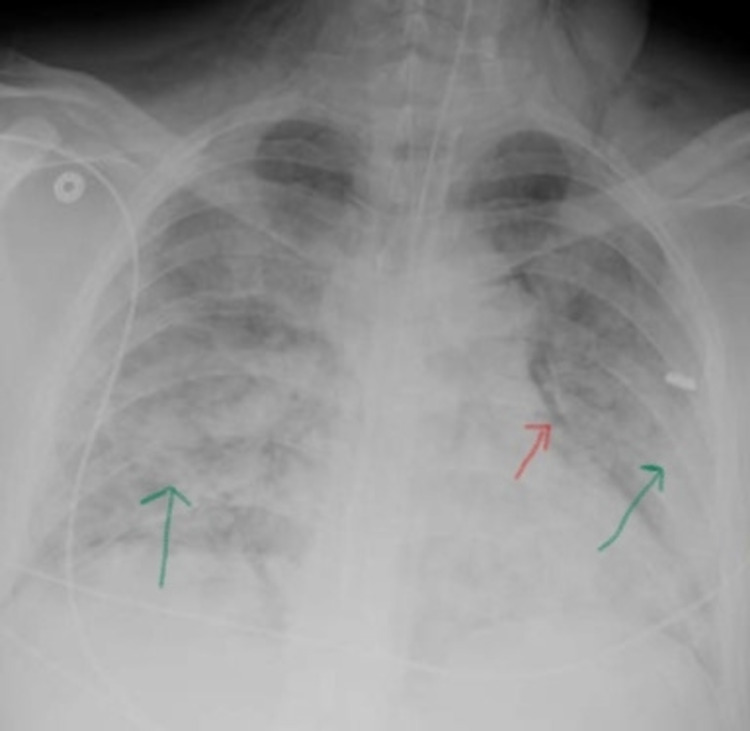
X-ray chest showing pneumomediastinum (red arrow) and bilateral extensive airspace opacifications (green arrows)
